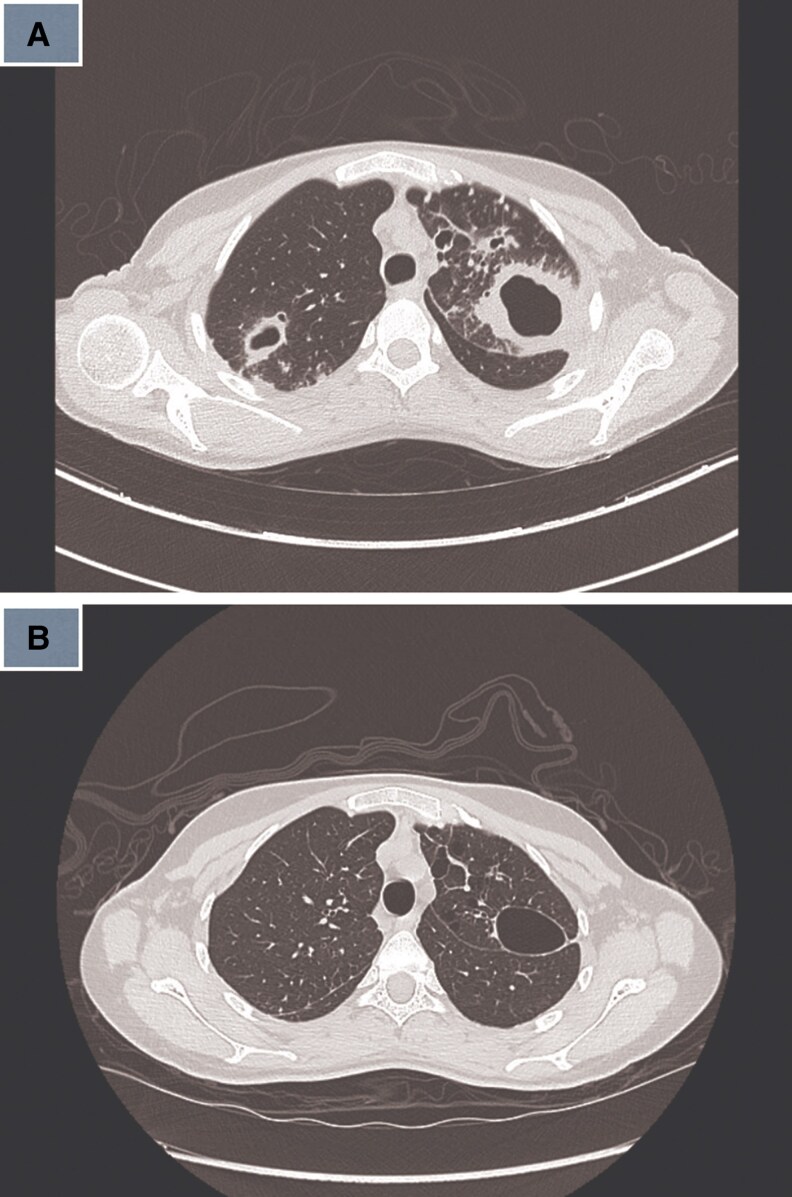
(*A*) Radiological image (eg, CT scan) showing cavitary lesions and consolidation in a pulmonary *M riyadhense* infection (case 5; Table 2). (*B*) Follow-up imaging showing resolution of lesions posttreatment (case 5; Table 2).
